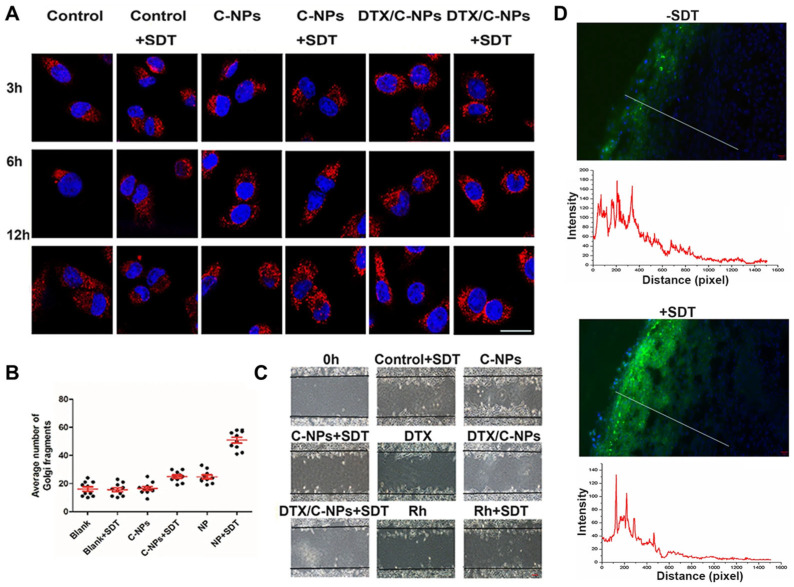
(A) Integrity of Golgi apparatus structure incubated with different preparations with/without SDT treatment. (B) Fragmented quantitation in Golgi apparatus for A549 cells after treatments. (C) Wound healing ability of A549 cells incubated in the absence and presence of SDT for 24 h. (D) Fluorescence distribution and intensity of NPs in A549 solid tumor with/without ultrasound treatment. Reproduced from [121].
